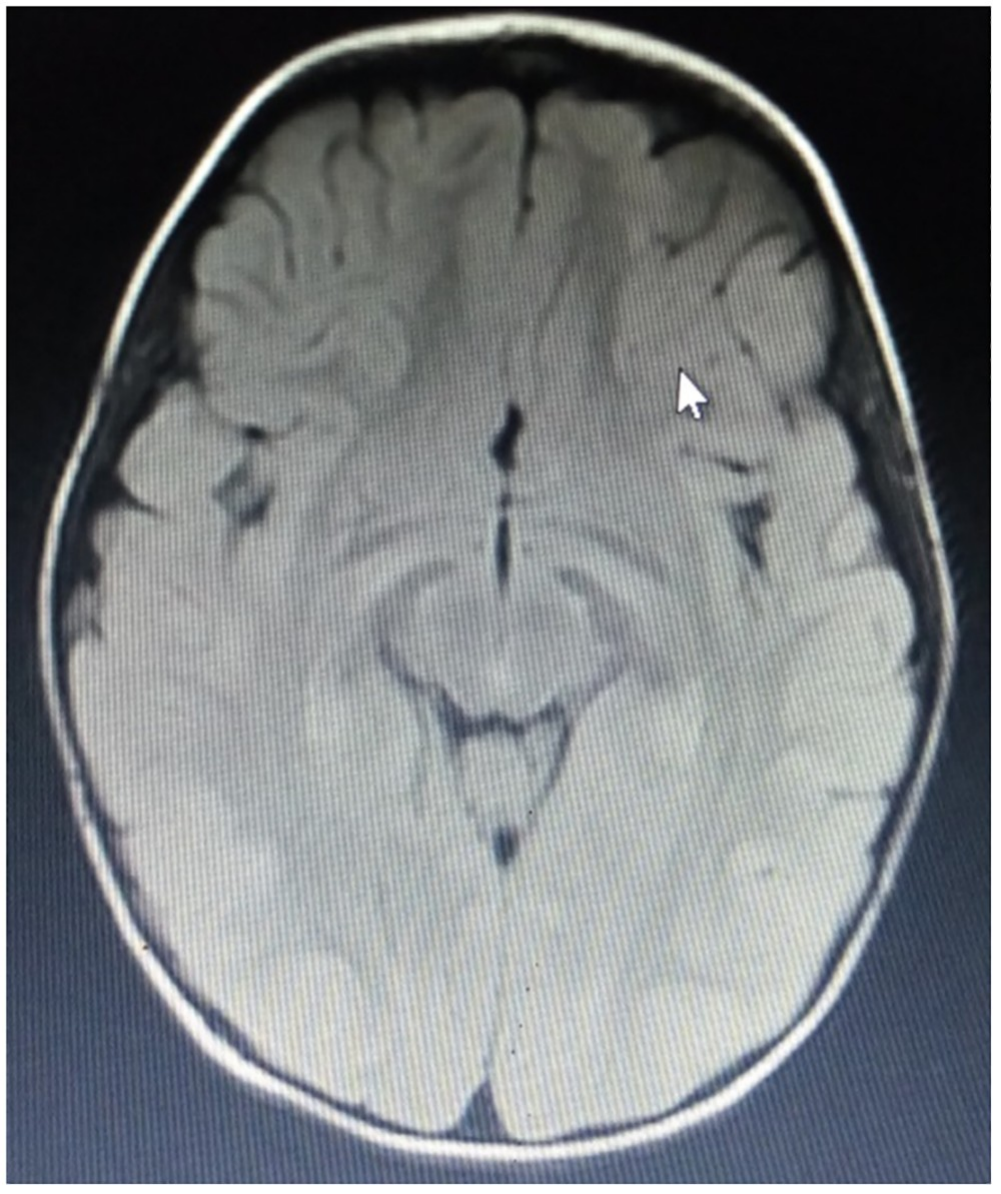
Case 30: MRI of a 3 year old patient with choreoathetoid CP. MRI of a 3 year old female patient born at term presenting with choreoathetoid CP, showing bilateral frontal lobe volume loss compared with the temporal-parietal and occipital formations and Bilateral basal ganglia posterior hyperintensity on axial T2 FLAIR.
